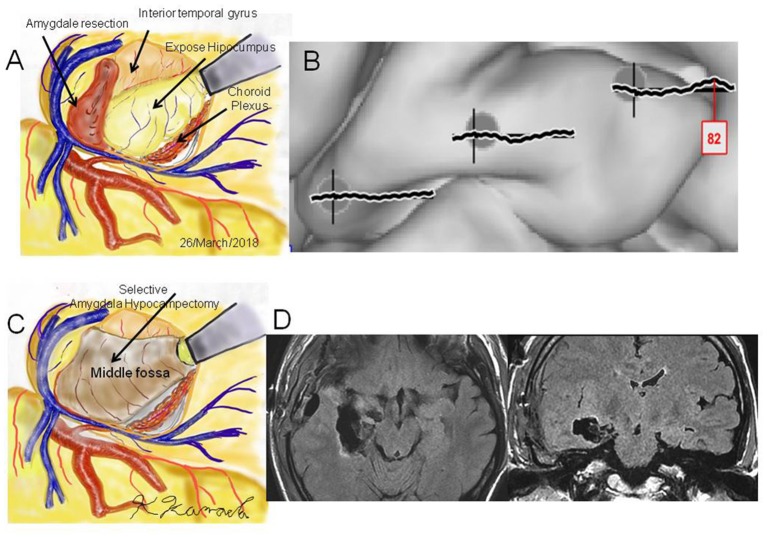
Case 2: (A) Operation sketch of disconnection of the Uncinate fascicles and removal of the anterior and medial roofs of the temporal horn. The hippocampus and choroid plexus were widely exposed. (B) Intraoperative cortico-cortico evoked potentials becoming extinct by hippocampal stimulation. (C) Operation sketch of complete hippocampectomy. (D) Postoperative magnetic resonance imaging showing selective hippocampectomy *via* the anterior ventral roof.
